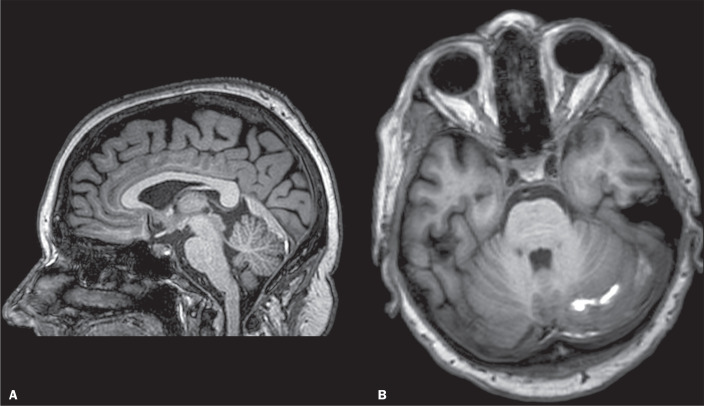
Sagittal T1-weighted image (A) showing a thrombus in the straight sinus and axial T1-weighted image (B) showing a linear hyperintense signal among the cerebellar folia. The final diagnosis was straight sinus thrombosis with subarachnoid hemorrhage and subdural hematoma.
